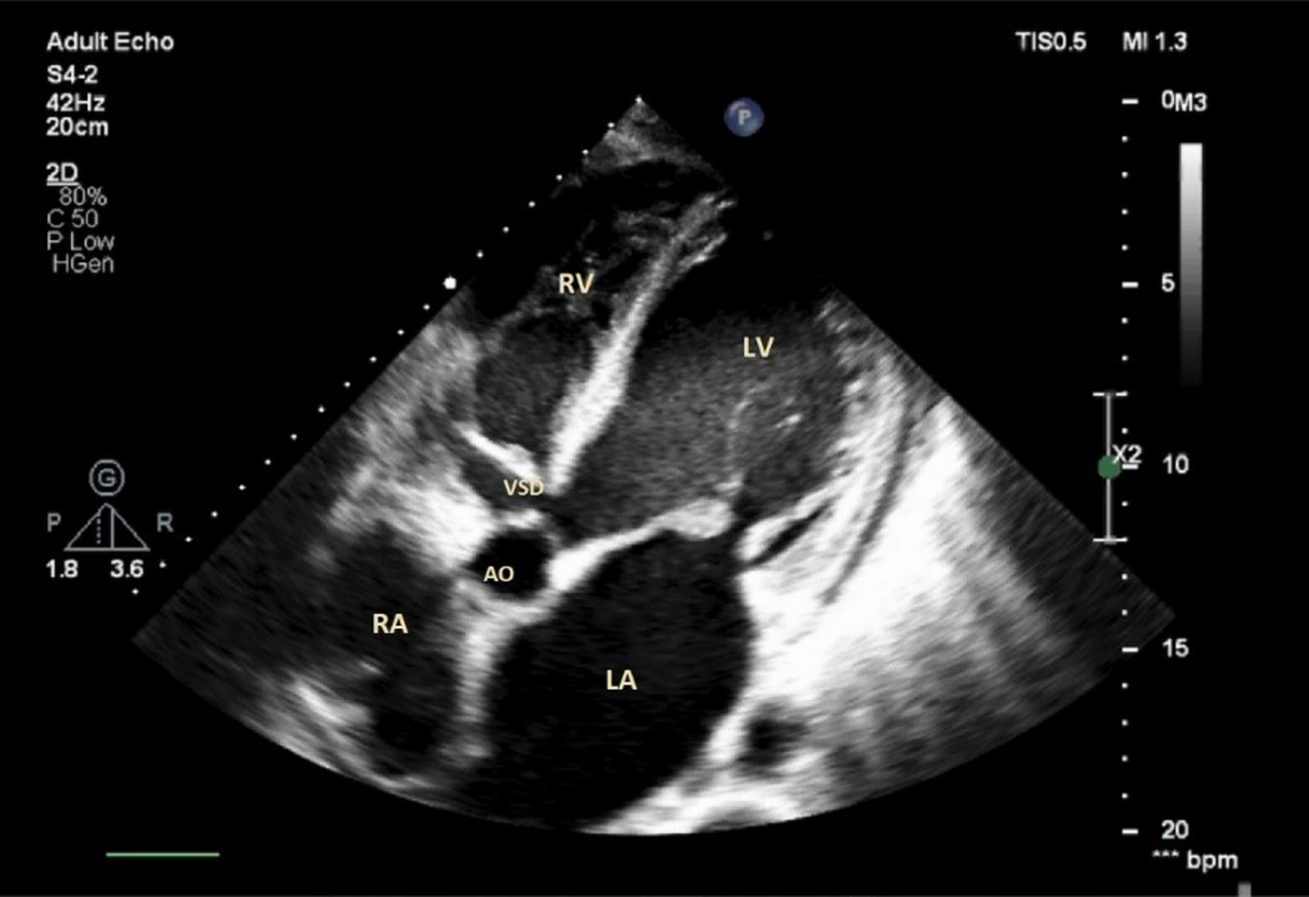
Transthoracic echocardiography in off‐axis view shows a tunnel shape VSD. LA, left atrium; LV, left ventricle; RA, right atrium; RV, right ventricle; VSD, ventricular septal defect.
